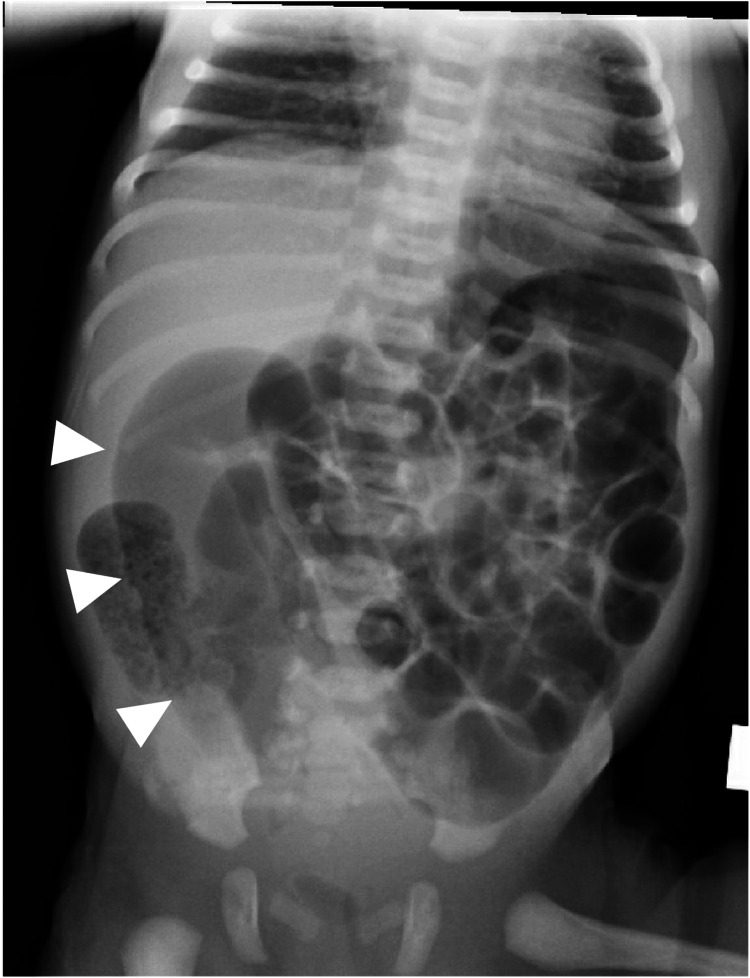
Abdominal X-ray. Multiple dilated bowel loops with an S-shaped dilated loop of bowel traveling downward toward the pelvis representing the sigmoid colon (white arrowheads).
